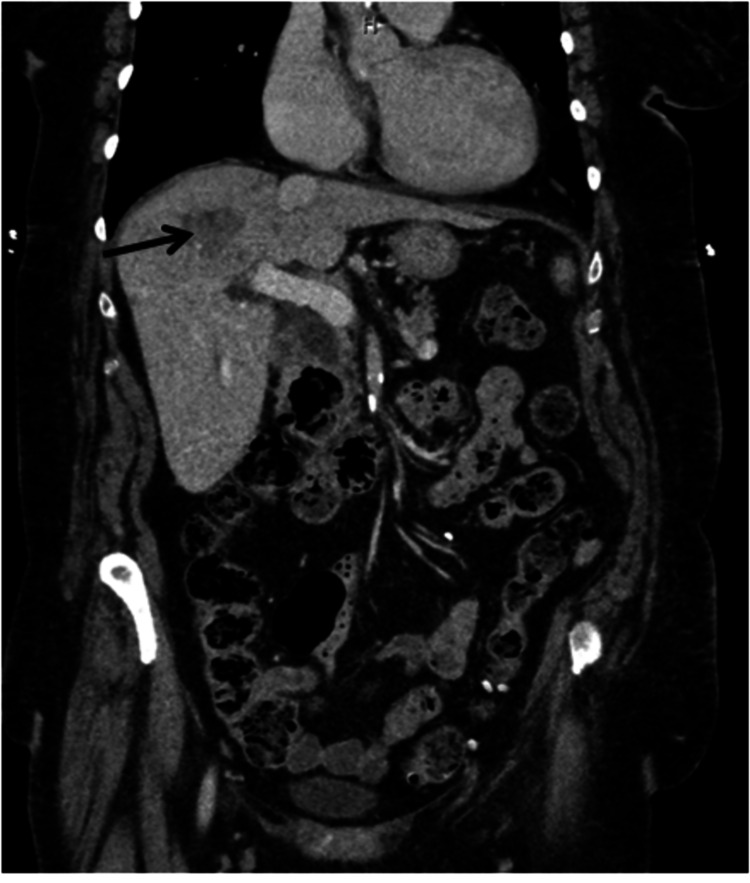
Computed tomography scan abdomen: poorly defined 3.4 cm × 2.3 cm hypoattenuating region with no peripheral enhancing lesion in the right hepatic lobe, indicating a hepatic abscess
